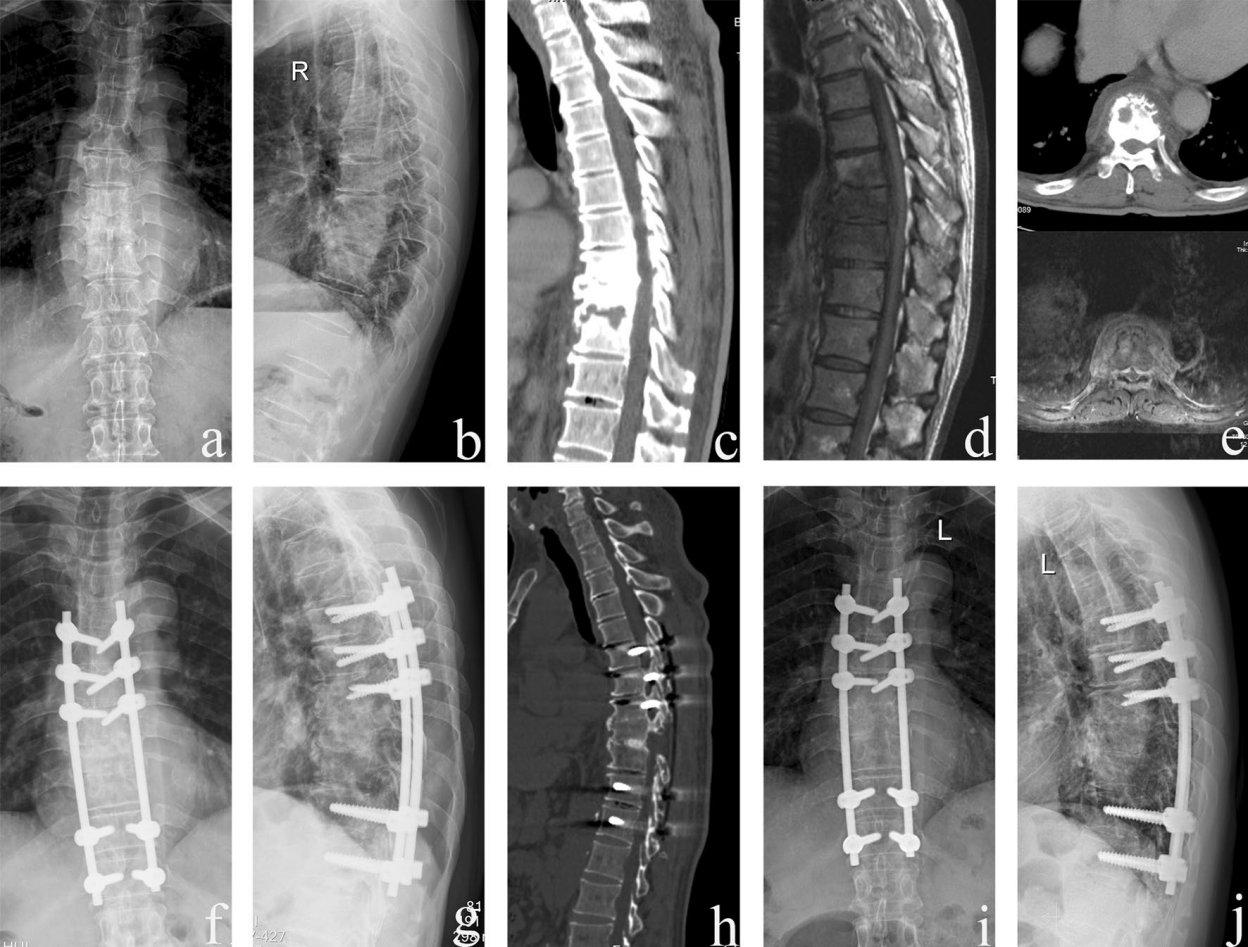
A 57-year-old male received a one-stage posterior transforaminal thoracic debridement, interbody fusion, and fixation. (a–e) Preoperative radiographs, CT, and MRI scans showed T8–T10 vertebral bone destruction, intervertebral space narrowing and paravertebral abscess formation. (f,g) Postoperative radiographs demonstrated that internal fixation was achieved in a good position. (h) CT showed that solid bone fusion was obtained at 12 months after surgery. (i,j) Radiographs displayed good internal fixation position and solid bone fusion, with no loss of correction after 66 months follow-up.
